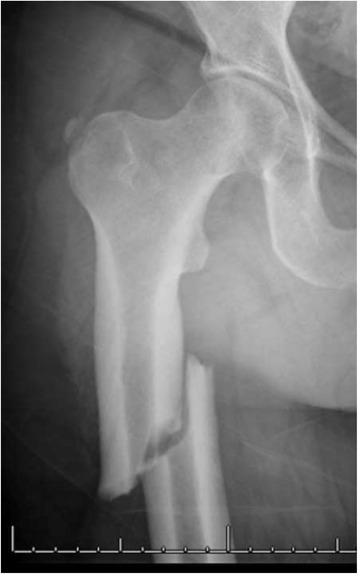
Radiograph of a patient with a complete AFF. Note the substantially transverse orientation of the fracture line at the lateral cortex, the medial spike and the generalized cortical thickening
